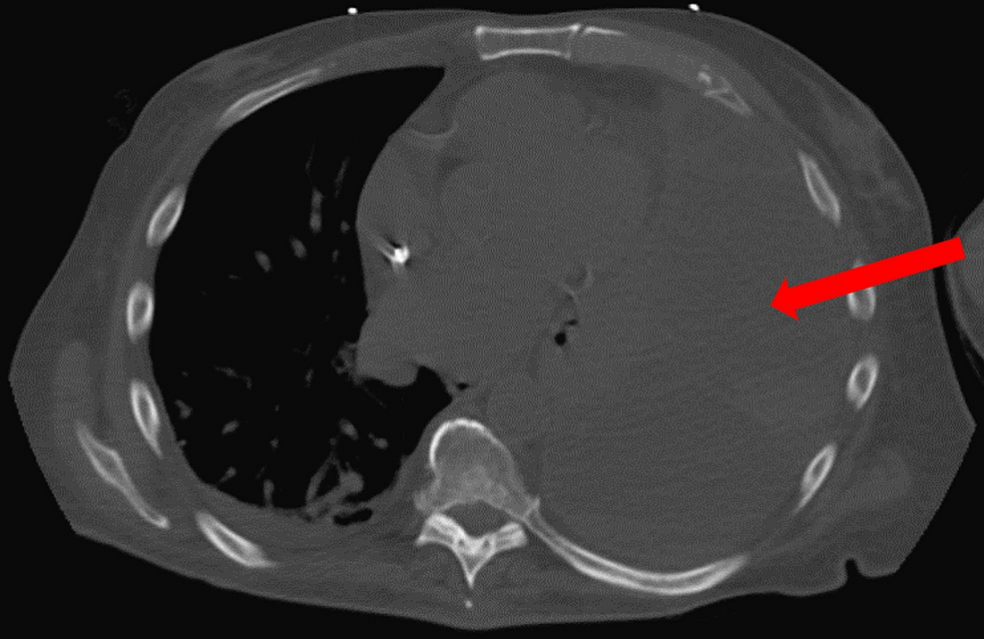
CT scan of the chest showing massive left-sided pleural effusion (red arrow). CT: computed tomography
